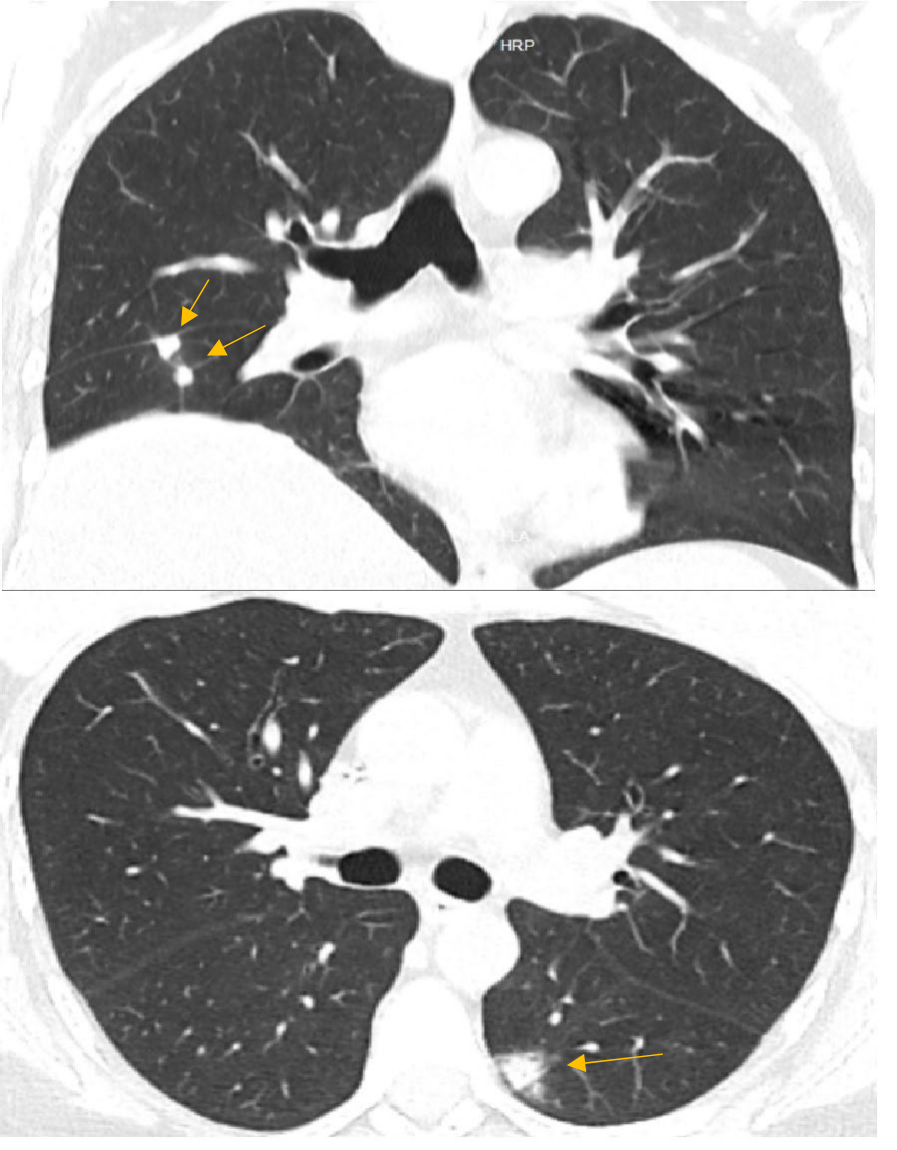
High resolution chest computed tomography showing bilateral solid nodules with ground glass halo (arrows). Source: Authors
